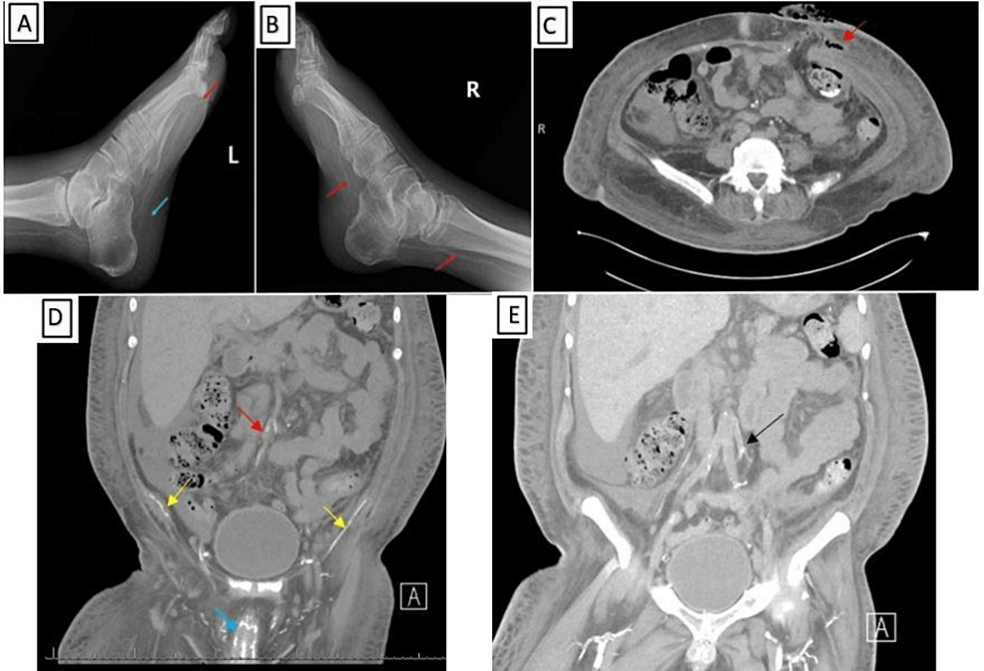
Radiological findings A and B: Left and right foot X-rays showing calcification of blood vessels (red arrows) and capillaries with reticular pattern within the soft tissue (blue arrow). C: CT scan of the abdomen and pelvis (axial) showing gas in the soft tissue (red arrow) at the site of colostomy lip. D and E: CT of the abdomen and pelvis (coronal) showing abdominal vessel calcification (red arrow), pelvis vessel calcification (yellow arrow), penile vessel calcification (blue arrow), and lead pipe calcification of the inferior mesenteric artery (black arrow). CT: computed tomography
